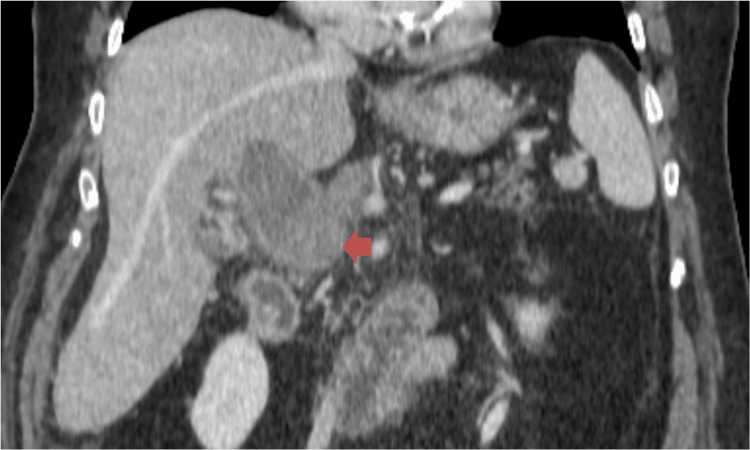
Coronal abdominal CT image highlighting the PVT (red arrow) PVT, portal vein thrombosis
